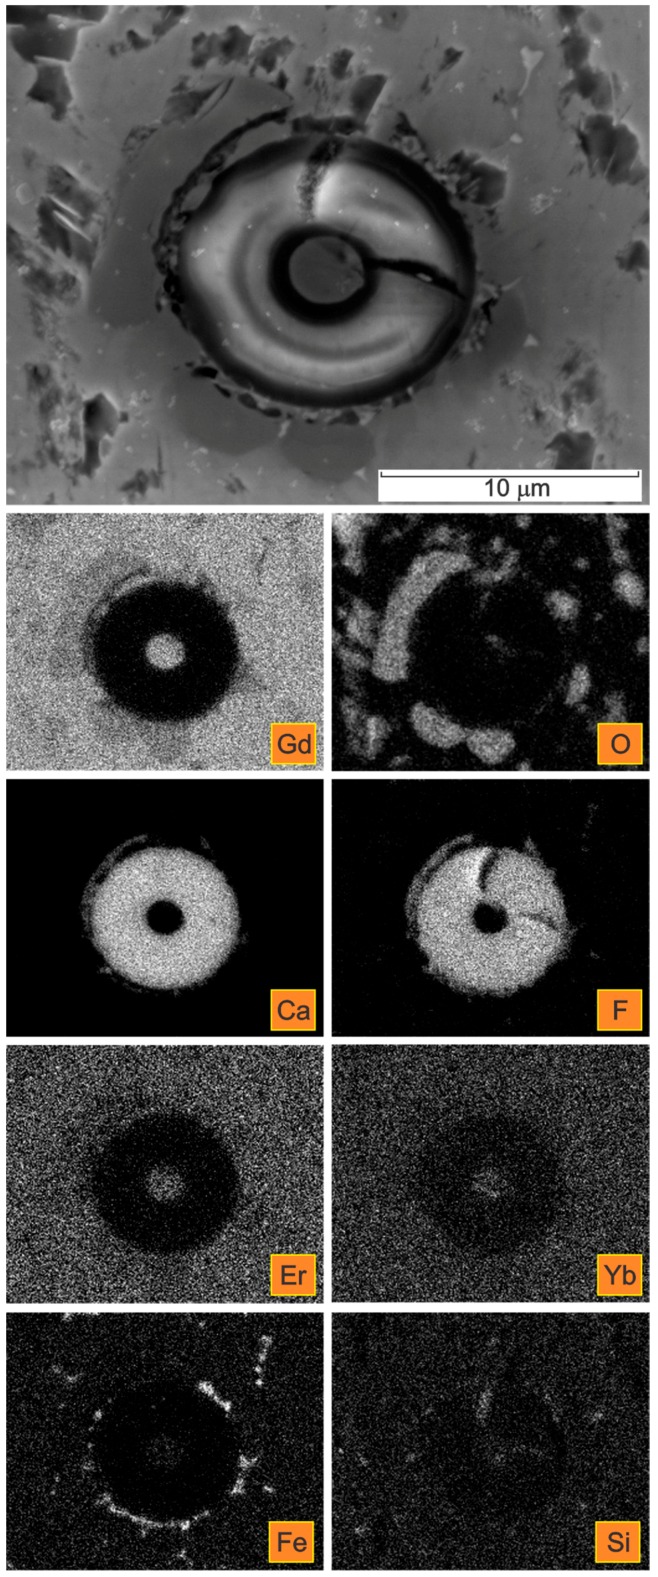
SE image of a CaF inclusion in a Gd cylinder in the extrusion transversal direction with the corresponding X-ray elemental mappings.
